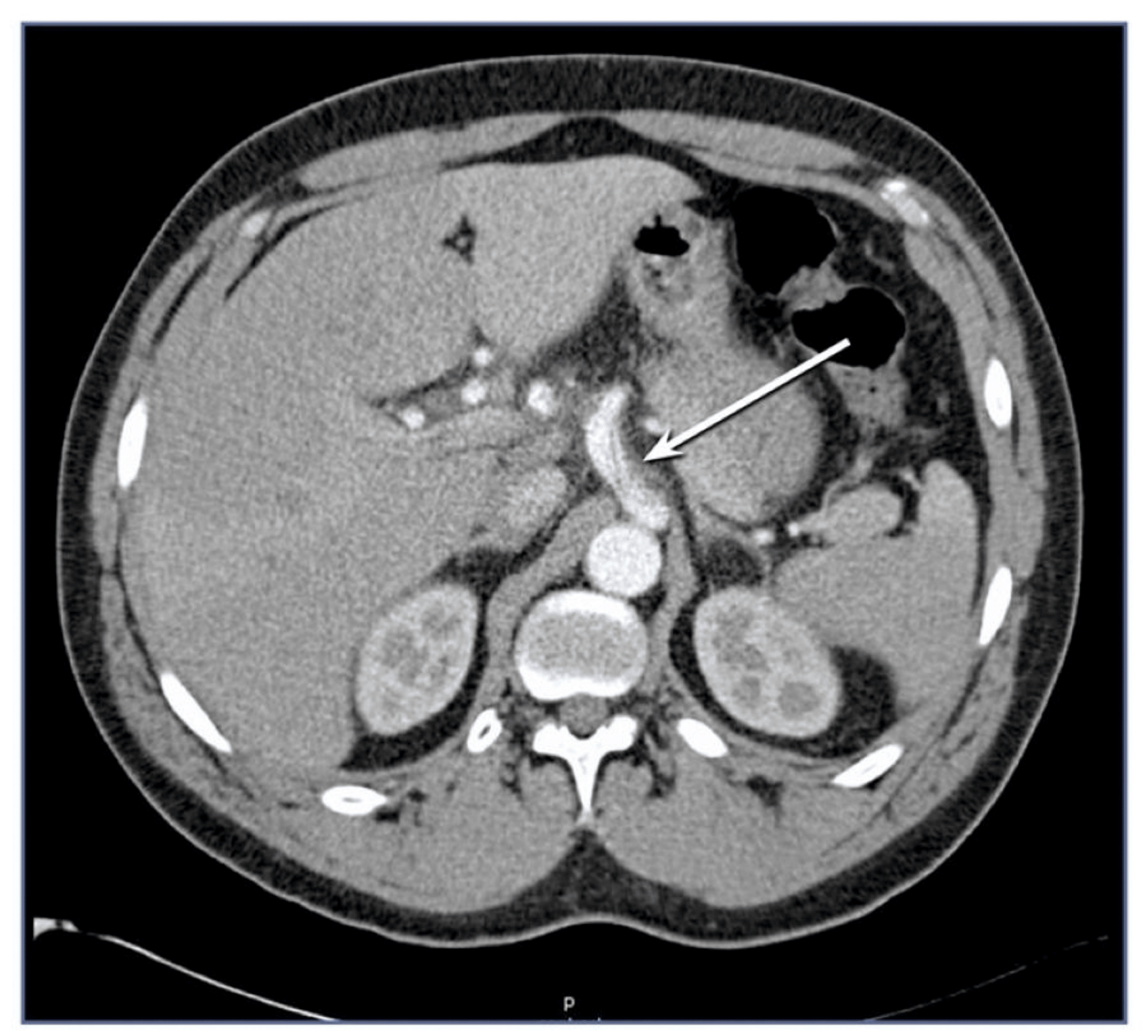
CT of the abdomen in the axial view shows the celiac trunk = level of L1 (white arrow).
